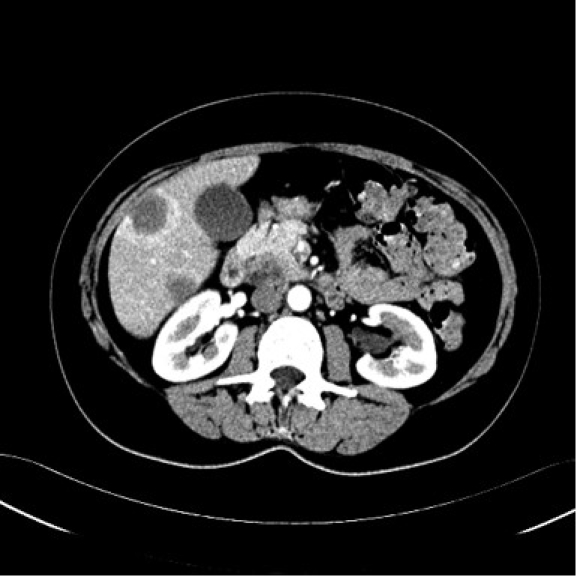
Abdominal computed tomography scan with contrast during the arterial phase. Multiple low–
